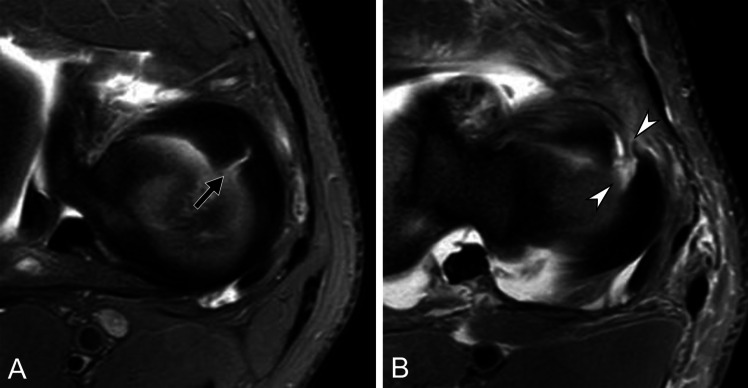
Partial versus complete radial tears. Radial tears of the anterior horn and body junction of the lateral meniscus in two different patents with ACL rupture depicted on axial proton-density-weighted fat-suppressed MRI (A, B). A 27-year-old man with incomplete, or partial-width, radial tear of the lateral meniscus (arrow, A) that spares the peripheral third of the meniscus. A 17-year-old man with complete, or full-width, radial tear of the lateral meniscus extending from free edge to periphery (arrowheads, B) with 5 mm gap
